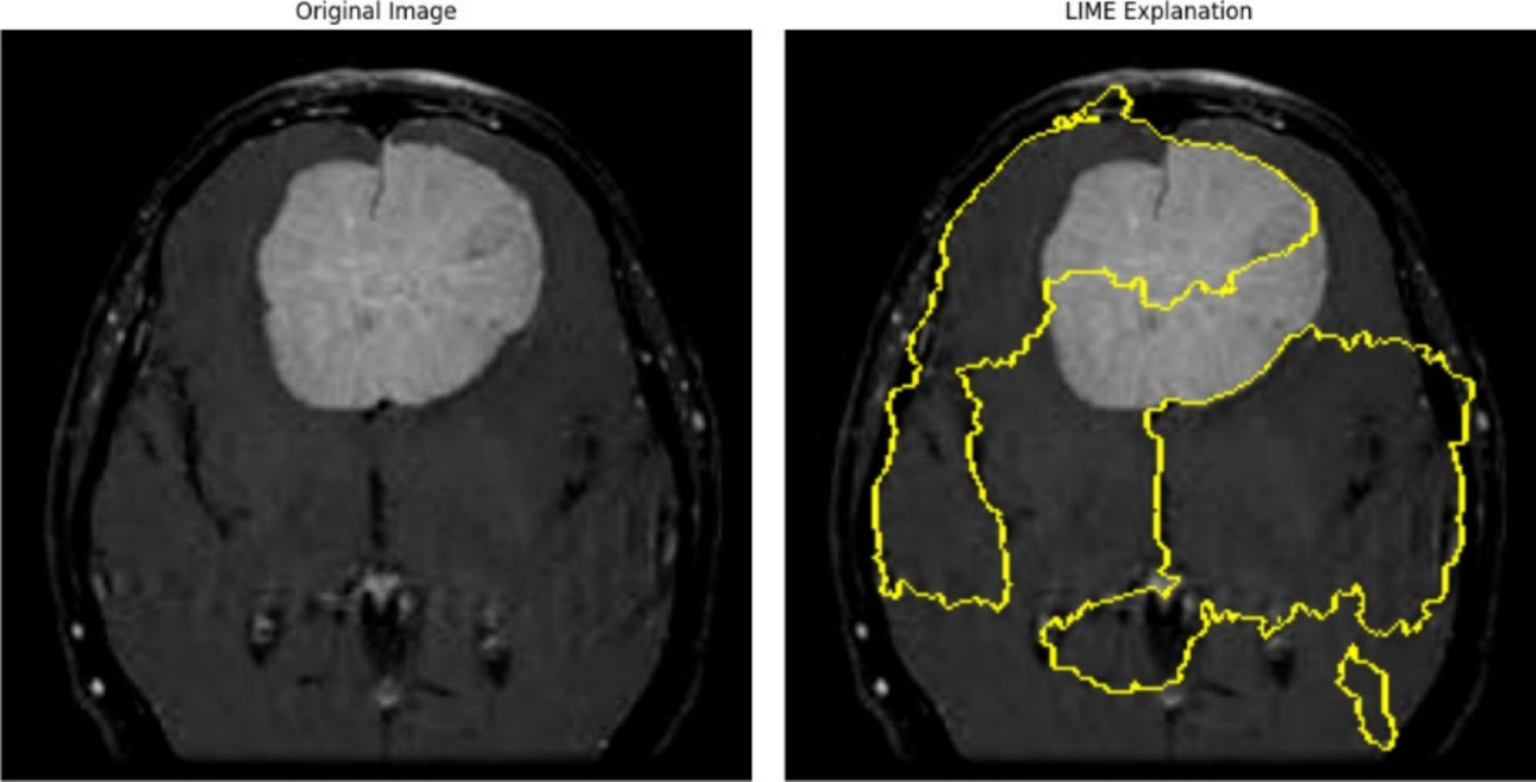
Lime visualization of glioma MRI image in densenet50 layers.
